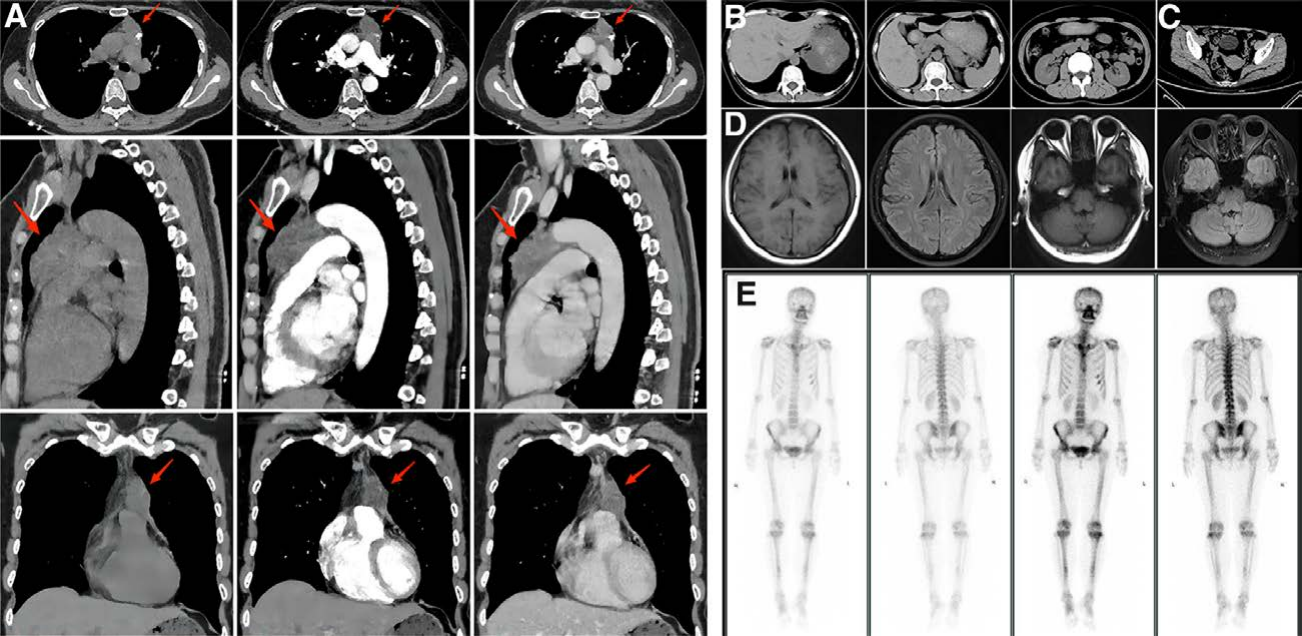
Radiological examination before surgery. (a) A huge irregular soft tissue density shadow (the red arrow) measuring 4.8 * 2.5 cm in size was observed in the anterior mediastinum by chest CT, with uneven enhancement in the arterial and venous phase. (b‐d) There was normal in abdominal CT, pelvic CT and brain MRI. (e) Whole-body bone ECT examination revealed that partial bone metabolism was slightly active, which indicated the benign disease.
